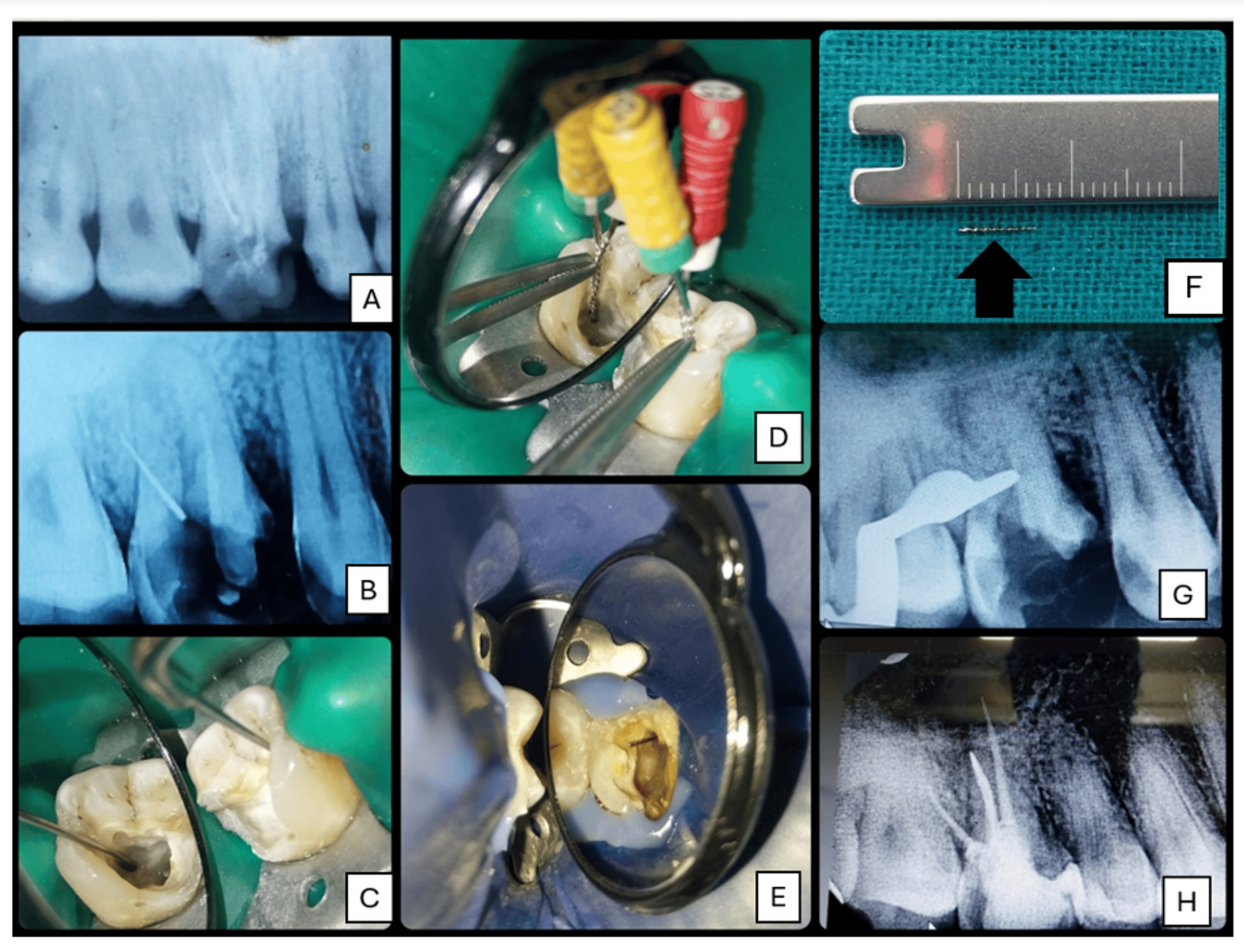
File retrieval from tooth 16 using ultrasonics and braiding technique. (A) Pre-op IOPA. (B) IOPA post-gutta-percha removal and confirmation of the separated instrument in the distobuccal canal. (C) Use of ultrasonic cutting tip T1 and non-cutting tip T3 for access refinement. (D) Braiding technique with H-files #20 and #25. (E) Retrieved instrument visible in chamber. (F) Retrieved instrument for scale (approx. 7 mm). (G) IOPA confirming complete file retrieval and patency. (H) Post-obturation IOPA IOPA: intraoral periapical
